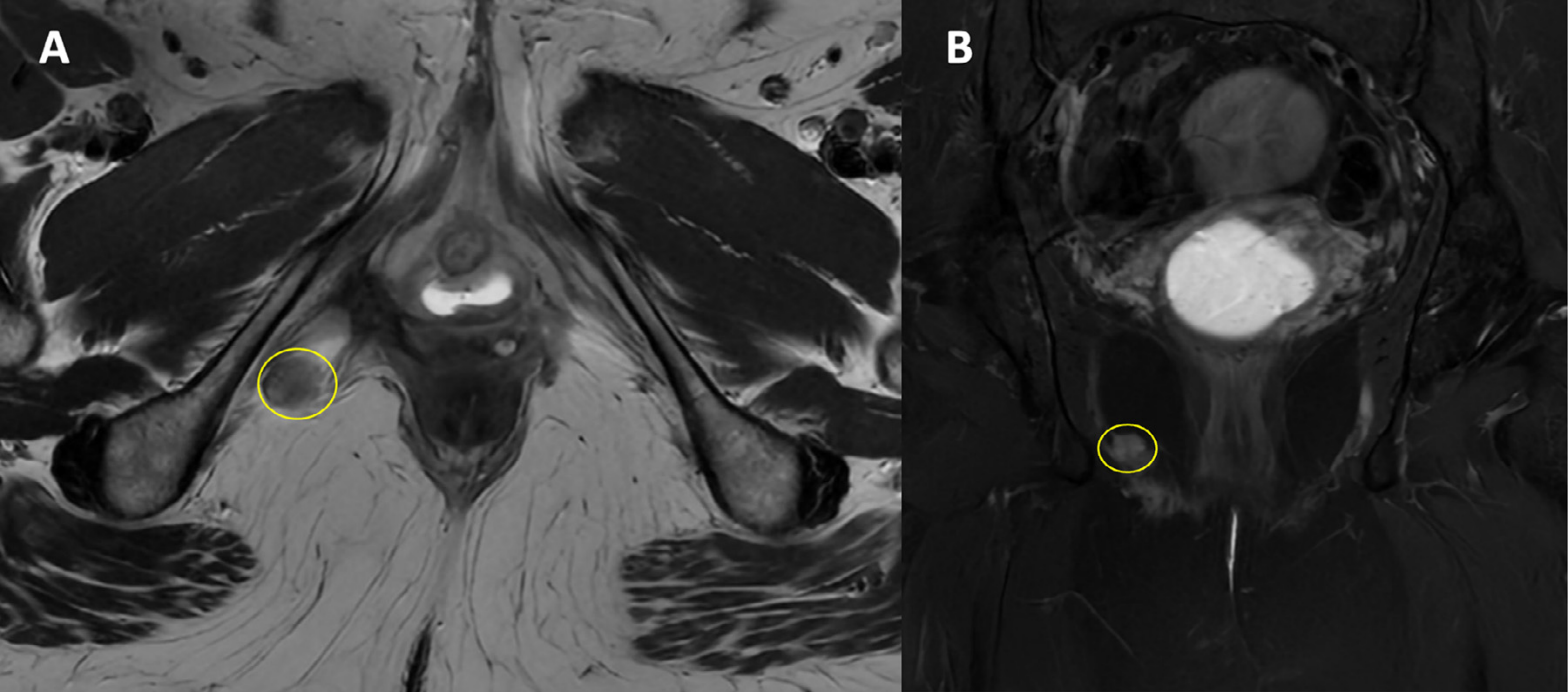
MRI scan T2w with gadolinium contrast, performed to assess for local recurrence, showed a 1 cm hyperintense lesion at the pudendal nerve route without invading the side wall, muscles and bones. May 2023, pre-SABR. (A, axial view T2w; B, coronal view, T2w Fat-suppressed).
